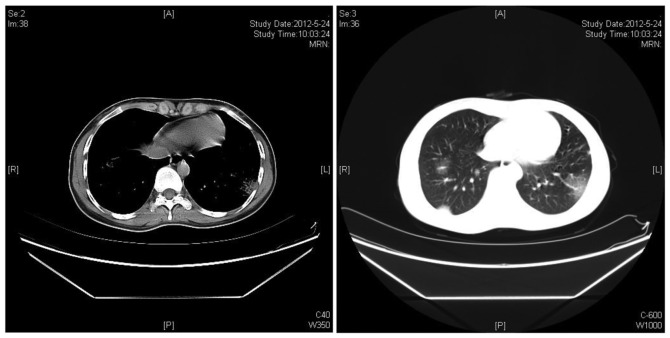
Computed tomography findings following 2 cycles of chemotherapy.
